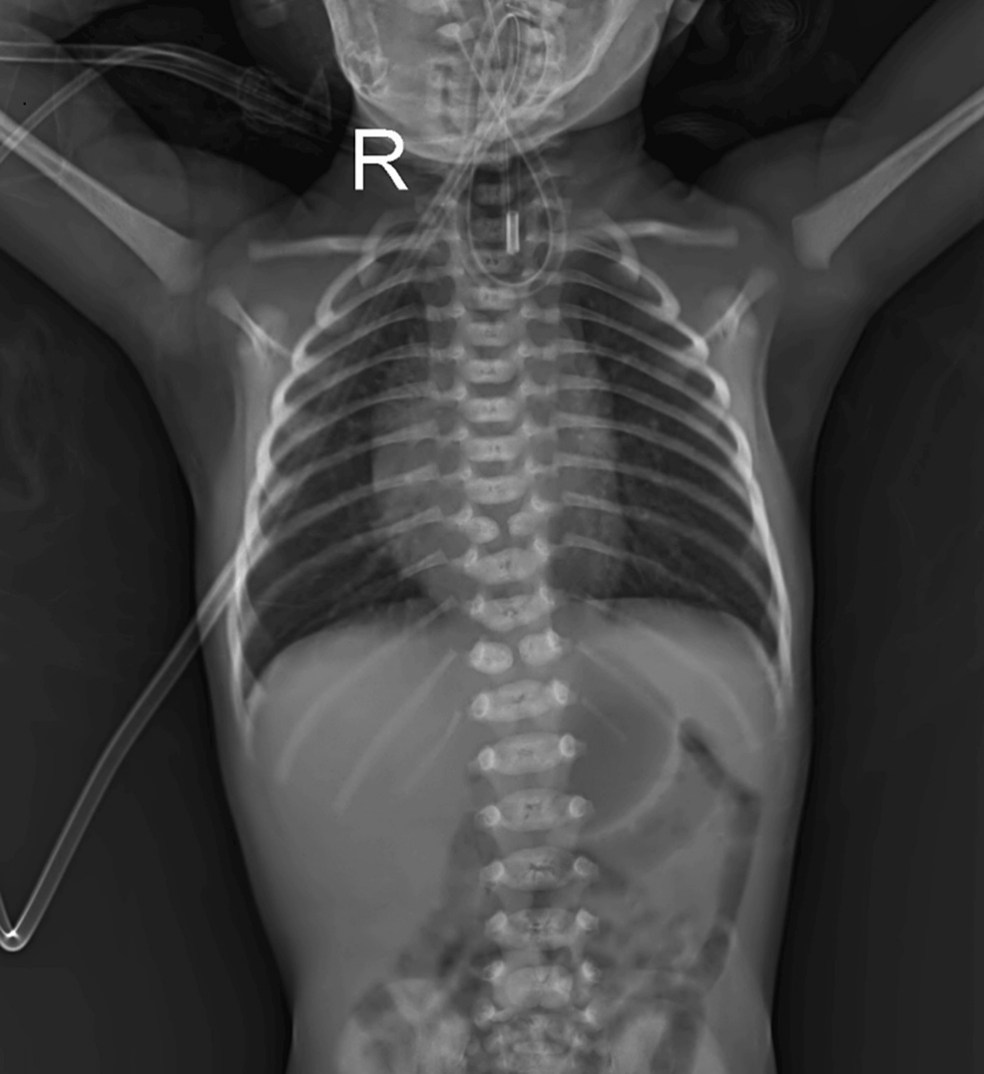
Preoperative X-ray imaging confirmed the diagnosis of a tracheoesophageal fistula
